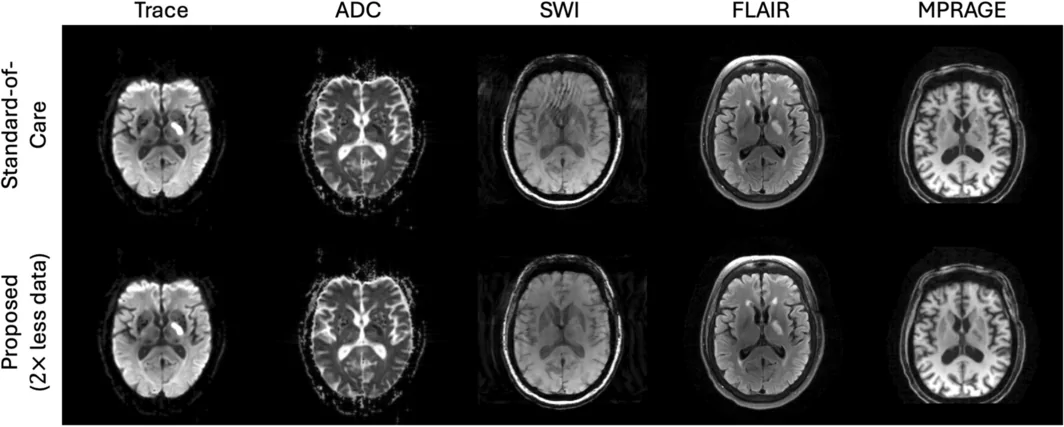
Representative images from a clinical stroke protocol are shown for a single subject, comparing standard‐of‐care reconstructions (top row) with images retrospectively under‐sampled by an additional factor of two and reconstructed using the proposed DPM‐based approach (bottom row). The protocol includes diffusion‐weighted imaging (shown as derived trace and apparent diffusion coefficient [ADC] maps), susceptibility‐weighted imaging [SWI], FLAIR, and MPRAGE. Across all contrasts, the proposed accelerated reconstructions preserve structural detail and contrast comparable to the standard‐of‐care. This subject was rated by Reader 1 (H.S.) in the blinded reader study, with the proposed reconstruction receiving the maximum score of 5 across all evaluated metrics and the standard‐of‐care reference receiving 5 on every metric except artifact level, where it received 4. This subject was a patient with ischemic stroke.
